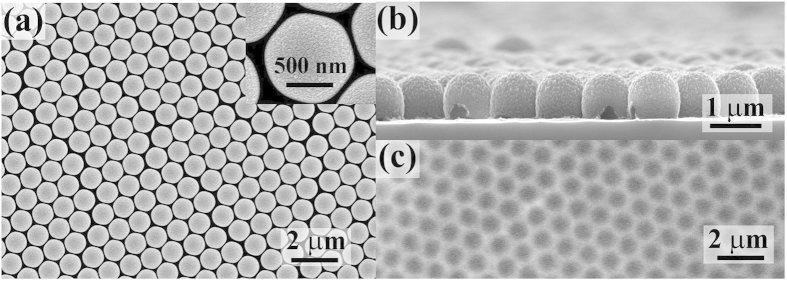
FE-SEM image of Co/Nb hybrid film based on 1 μm PSs template. (a) Top view, the inset is local magnification, (b) side view, (c) the interface of Co shells and Nb film after removing Co shells by ultrasonic cleaning.
